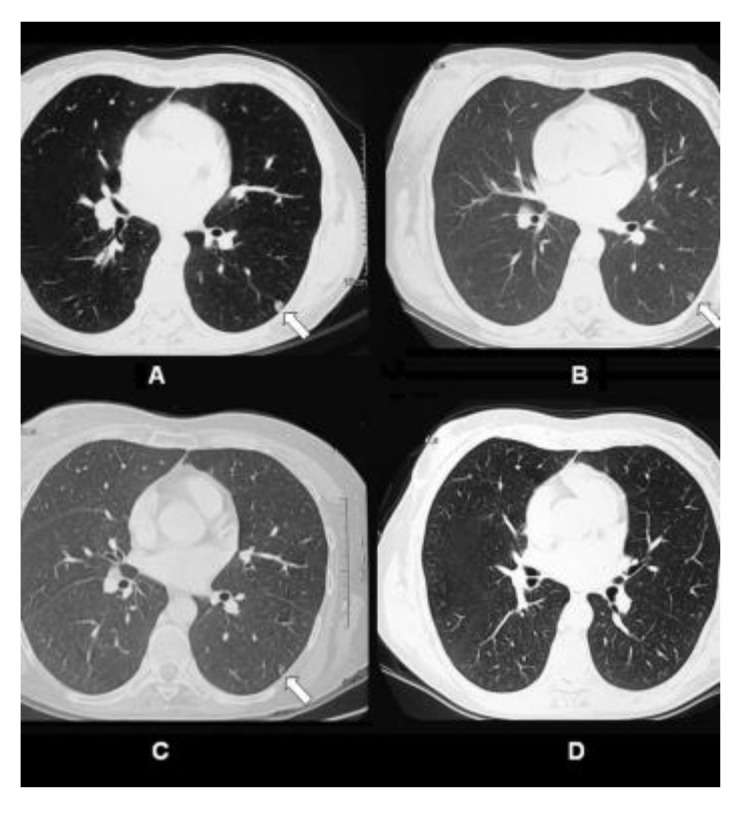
CT scans of pulmonary metastatic nodule in the left lung of 47-year-old female patient with stage IV breast cancer treated by laser ablation and IP-001 application. (A) CT scans of the patient taken before the first treatment. Small metastatic nodule was located in the left lung of the patient (indicated in arrow). (B) CT scans taken 1 week after the first treatment. (C) CT scans taken 2.5 months after the first treatment. (D) CT scans taken 12 months after the first treatment. This figure is reproduced from [35], with permission by the Royal Society of Chemistry and Owner Societies.
